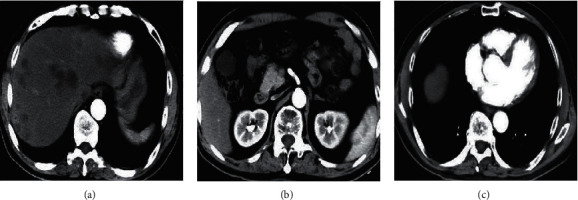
Preoperative CT diagnostic images of patients. (a) The image of a male patient aged 63 years. (b) The image of a female patient aged 58 years. (c) The image of a female patient aged 70 years.
